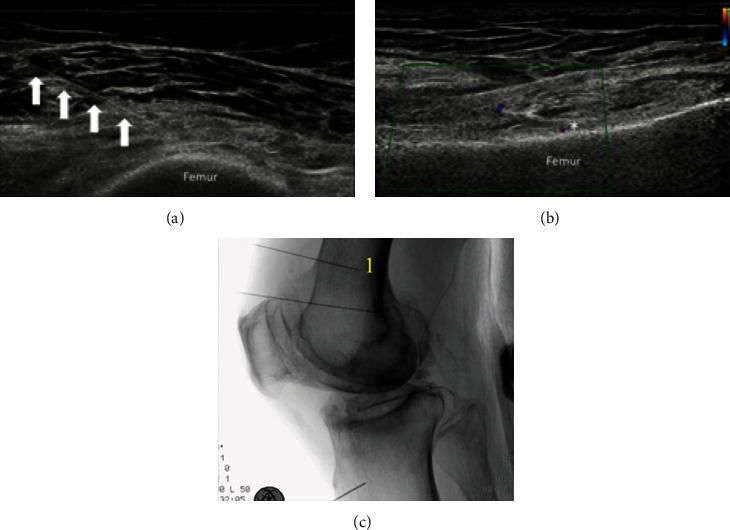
Nerve to vastus intermedius (NVI) denervation (either the medial or the lateral branches). (a) The transverse view shows the needle inserted in an in-plane approach to the midfemoral shaft. (b) The coronal view shows the Doppler signal that signifies superomedial or superolateral genicular artery at the junction of femoral shaft and femoral epicondyle. White arrow: needle; asterisk: the needle target. (c) A lateral view of fluoroscope of the knee with needle placement for NVI (either the medial or the lateral branches) denervation as shown at 1.
